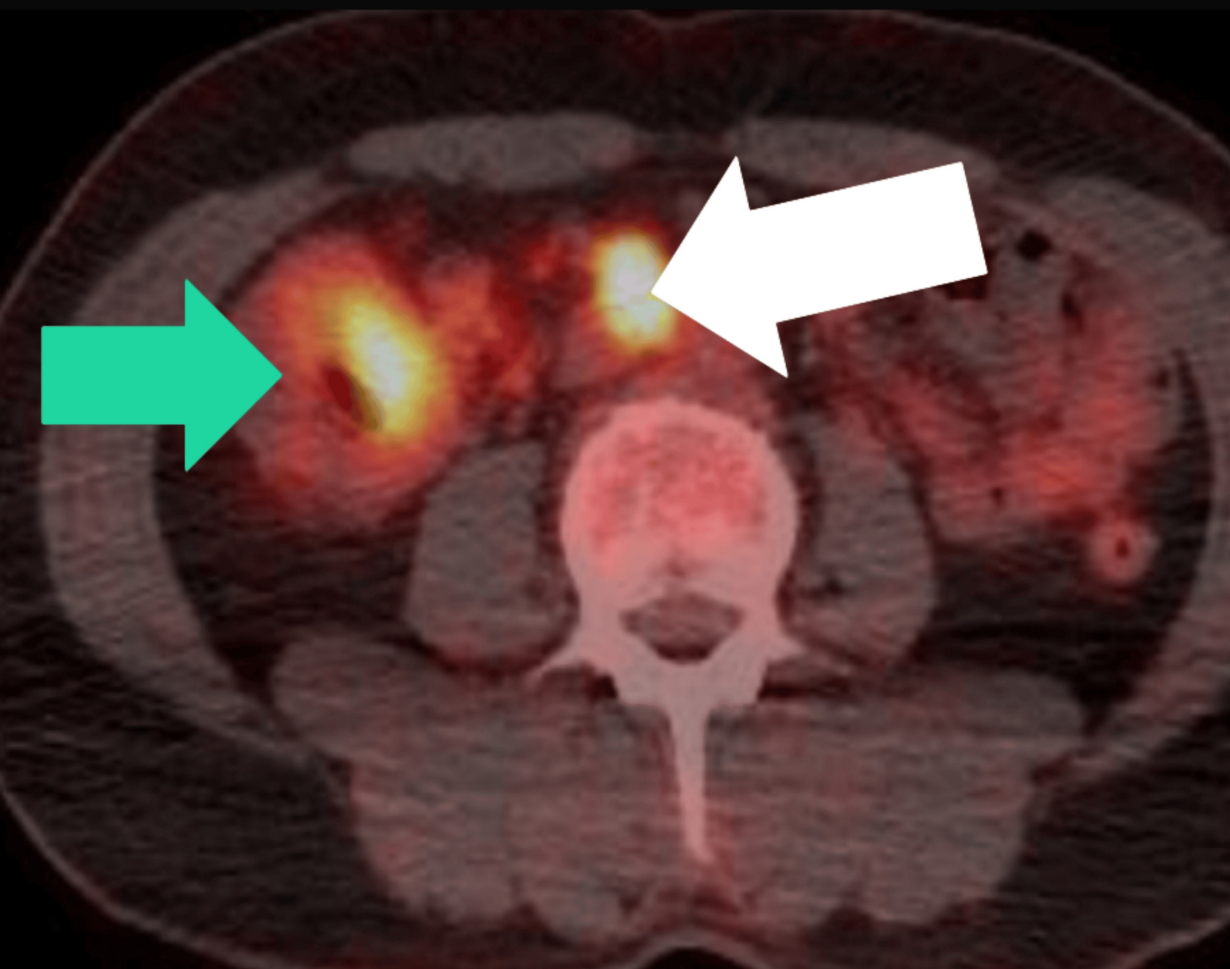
PET-CT image showing a hypermetabolic mass lesion localized to the ascending colon, as pointed by the green arrow, with evidence of distant metastasis with hypermetabolic lymph nodes, as pointed by the white arrow PET-CT: positron-emission tomography-computed tomography
